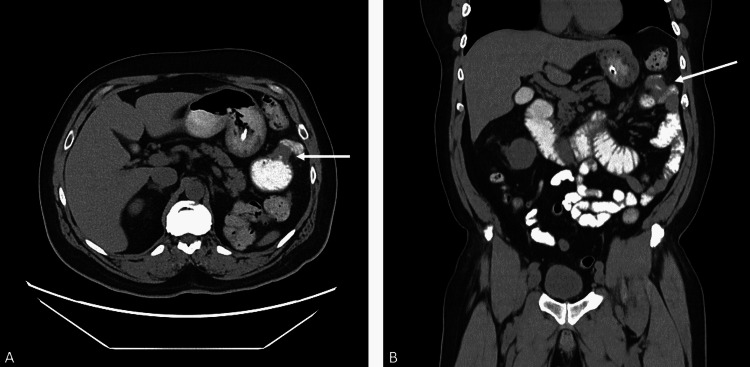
CT of the abdomen with barium sulfate contrast: (A) transverse plane and (B) coronal plane Focal narrowed segment of proximal to mid jejunum in the left upper quadrant responsible for partial bowel obstruction (arrows). Wall is thickened. The neoplastic disease is of concern, although the findings are nonspecific.
